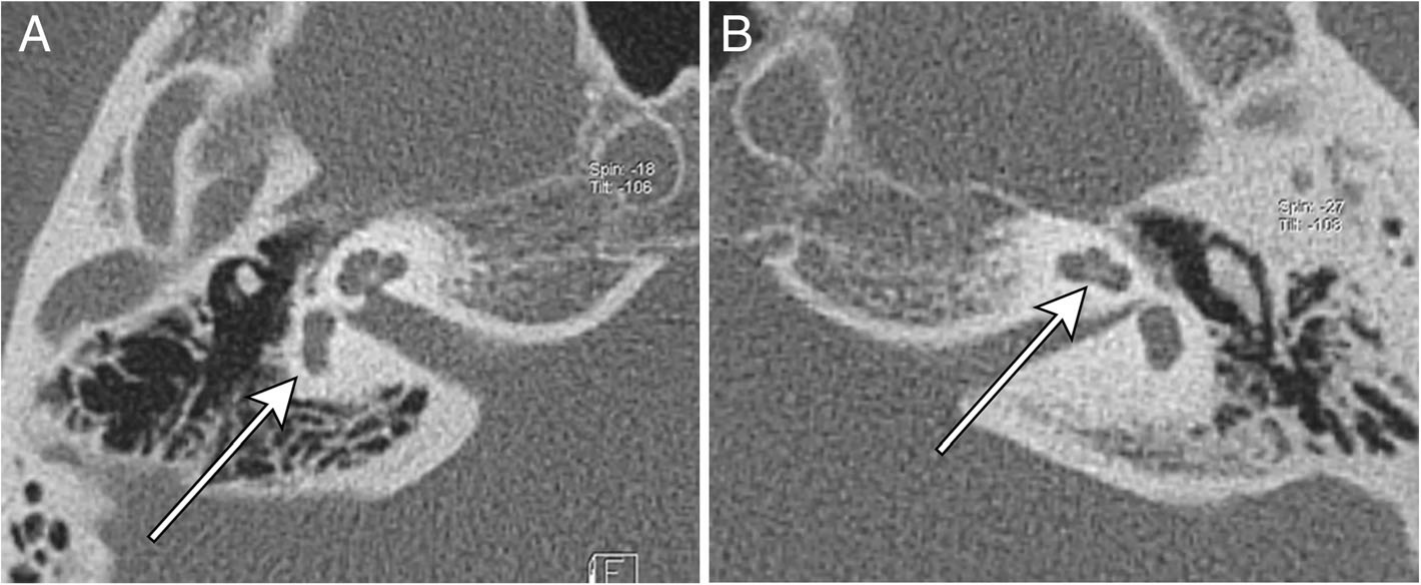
Temporal bone CT image showed aplasia of the semi-circular canals (a) and cochlear nerve canal atresia (b)
